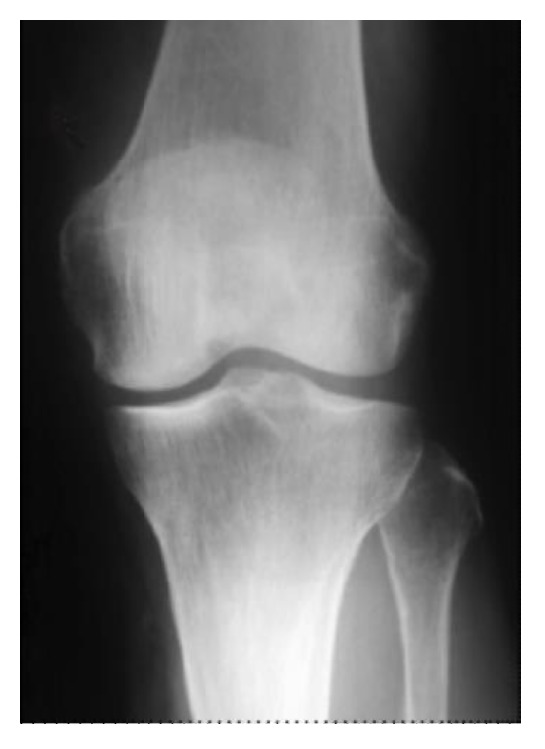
Case 1. Preoperative plain radiograph reveals no degenerative changes, suggesting Kellgren-Lawrence grade 1.
